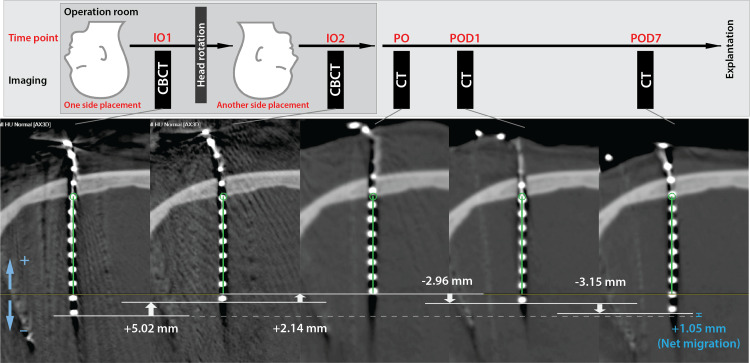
Study protocol and images from a representative patient who underwent bilateral stereotactic electrode implantation Upper low: Migration distance of each interval was used for analysis. For bilateral implantation, intraoperative CBCT was performed twice, once after implantation in each hemisphere. Lower low: Position of the depth electrode targeting the right parahippocampal gyrus at each time point. White arrows indicate migration distance. Negative values indicate electrode advancement (inward migration). CBCT: cone beam computed tomography; IO1: intraoperative image 1; IO2: intraoperative image 2; POD: postoperative day; PO: post-implantation
